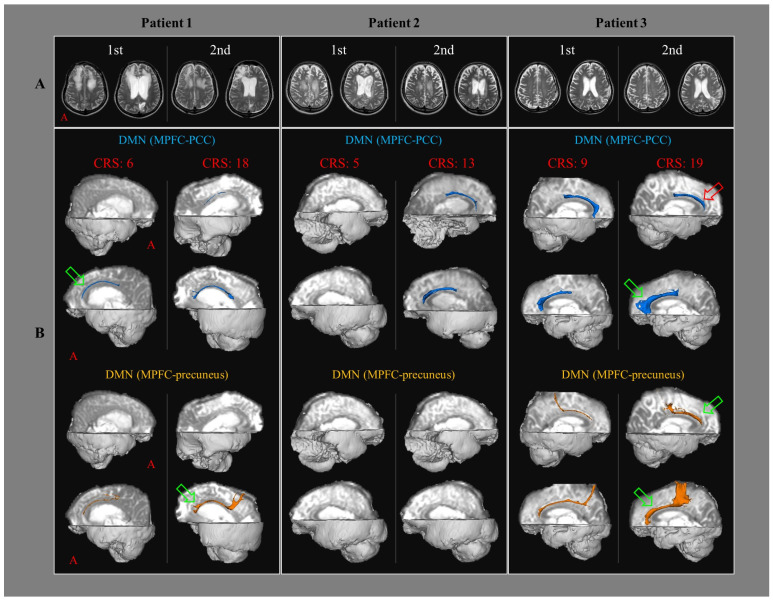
(A) The first and second T2-weighted brain magnetic resonance images of three patients of traumatic brain injury. (B) Diffusion tensor tractography (DTT) for the medial prefrontal cortex (mPFC)–posterior cingulate cortex (PCC) default mode network (DMN) and the mPFC–precuneus DMN. On the second DTT, reconstruction of the mPFC-PCC DMN is observed on the right side of patient 1 and both sides of patient 2, which were not reconstructed on the first DTT; in addition, thickening of the left side of patient 3 is observed (green arrows). However, narrowing is observed on the right side of patient 3 (red arrow). Thickening of the mPFC–precuneus DMN is observed on the left side of patient 1 and both sides of patient 3 (green arrows).
